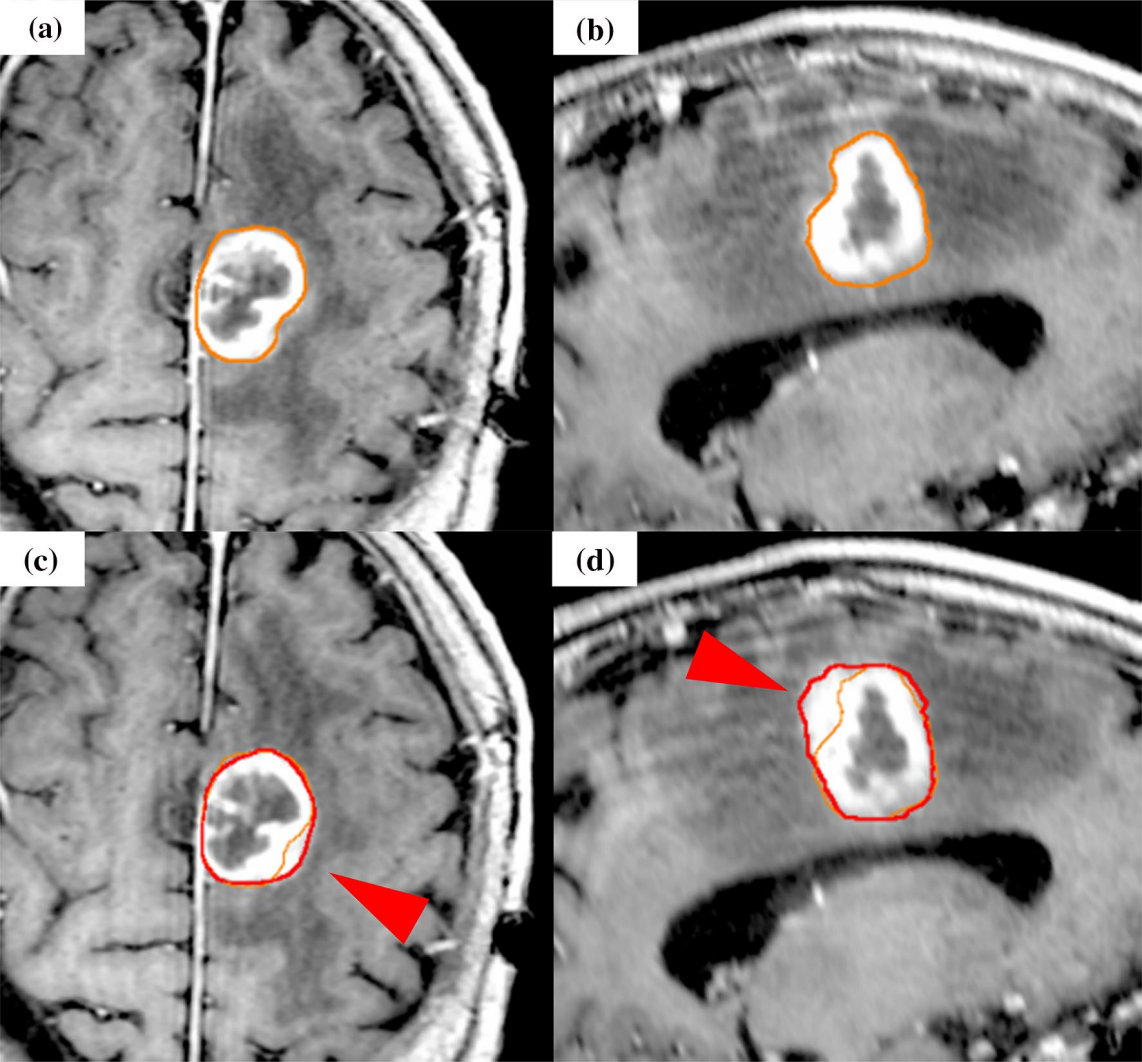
The case of shape change during stereotactic radiotherapy. The case was brain metastasis of which primary tumor was salivary gland pleomorphic adenoma. Orange line shows the gross tumor volume (GTV) of the initial plan, and red line shows GTV at the modification. A slight change of GTV delineation was observed (red arrow). a, b Axial, Sagittal: T1- weighted magnetic resonance imaging (MRI) with gadolinium (Gd) of the initial plan. c, d Axial, Sagittal: T1-weighted MRI with Gd before third treatment for the modification (the sixth day of SRT start)
